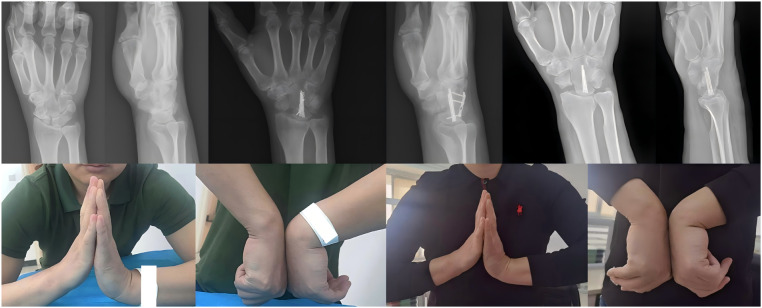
A typical case example. Preoperative and postoperative radiographs and clinical photographs.
